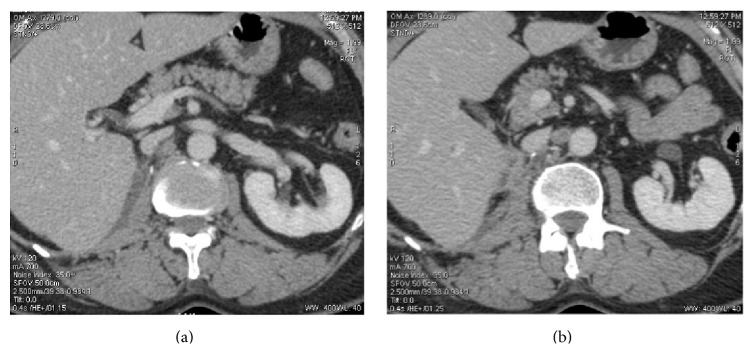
Postoperative CT scan. Axial enhanced CT scan showing (a) tissue thickening of 9 mm between the inferior vena cava and the right diaphragmatic pillar and (b) recent appearance of centimetric interaortocaval lymph node.
